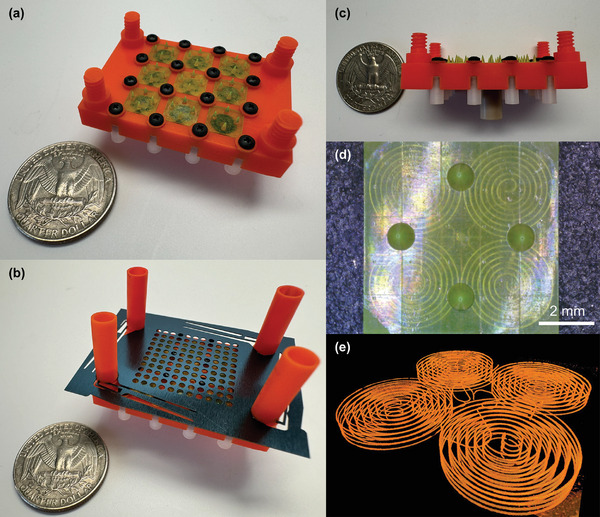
Images of a 3D‐printed electrospray thruster: a) optical image of the device without the extractor electrode next to a US quarter (the central emitter module was replaced with a non‐functional emitter module during the experimental characterization to ensure uniform emitter operation across the other eight emitter modules); b) optical image of a fully assembled device next to a US quarter; c) optical image from the side of the device without the extractor electrode next to a US quarter; d) close‐up optical image of an emitter module; e) X‐ray CT image of an emitter module, evidencing that the hydraulic network inside the module is free from resin.
